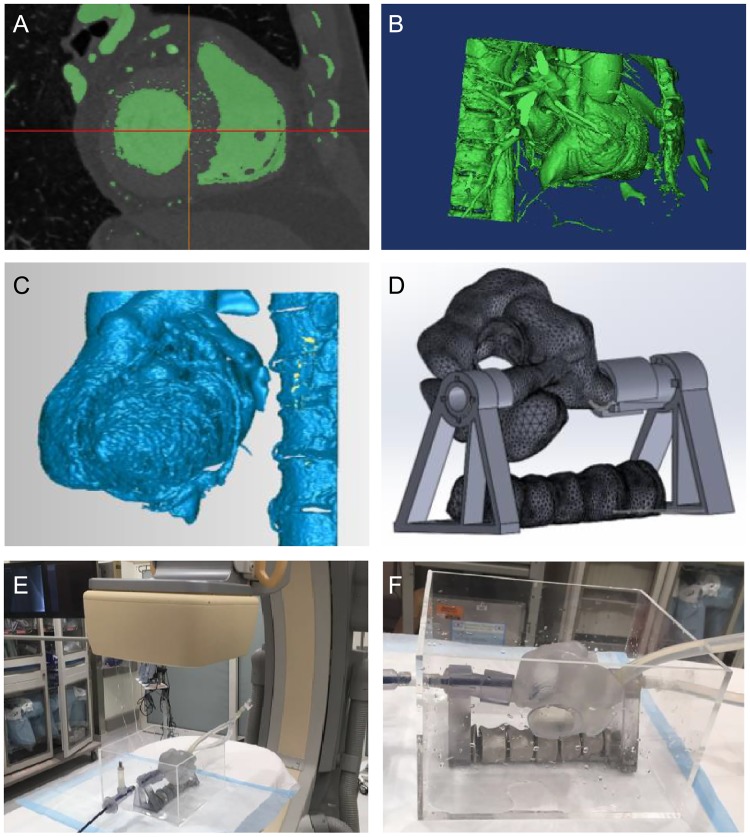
Segmentation of anatomical structures. A: A slice of CT scans shows the segmented region after thresholding. B: 3D reconstruction of the heart and spine by selecting the largest object and filling inner holes. C: The 3D models are cleaned by deleting peripheral blood vessels and bones. D: 3D models are further modified and fabricated using a 3D printer. E&F: The 3D printed model is tested under the X-ray fluoroscopy machine.
